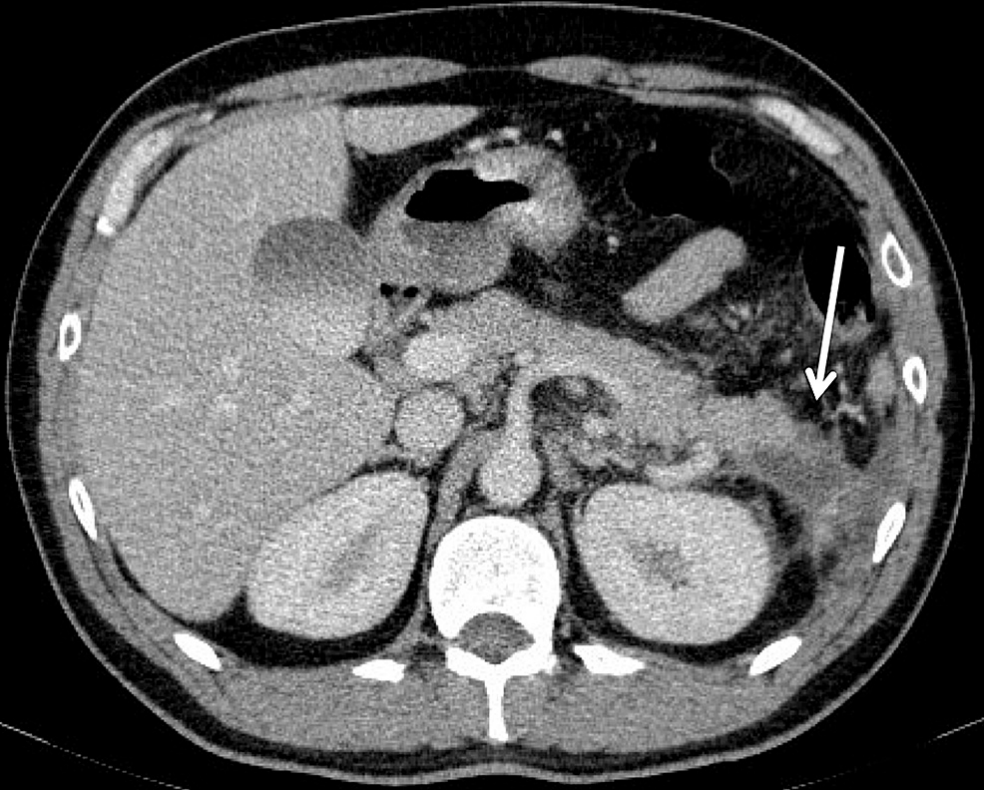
Contrast-enhanced CT scan (axial plane) on admission showing irregular margins on the tail of the pancreas in contact with a collection that extend into the anterior pararenal space.
